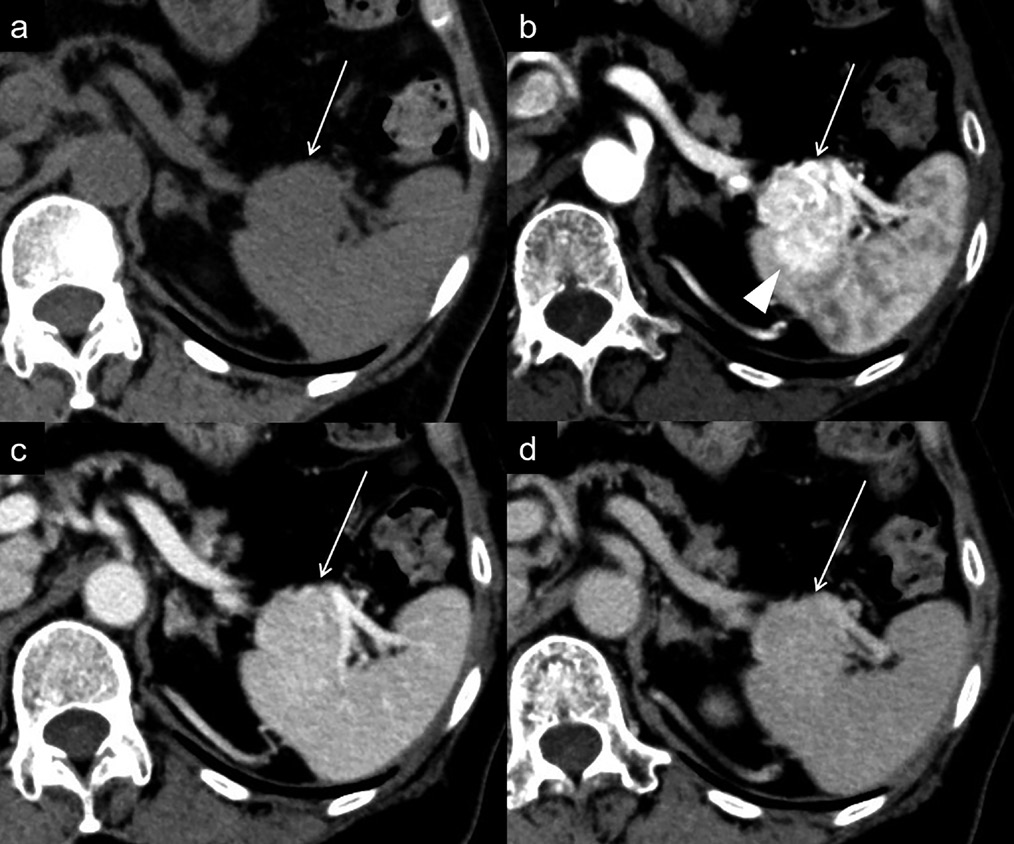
Dynamic contrast-enhanced CT (a. unenhanced phase, b. arterial phase, c. portal venous phase, d. equilibrium phase). A homogenous mass (a: arrow) is present in the tail of the pancreas. The mass shows heterogeneously marked enhancement in the arterial phase (b: arrow) and washout in the portal venous and equilibrium phases (c, d: arrow). The splenic invasion is clearly identified in the arterial phase (b: arrowhead).
